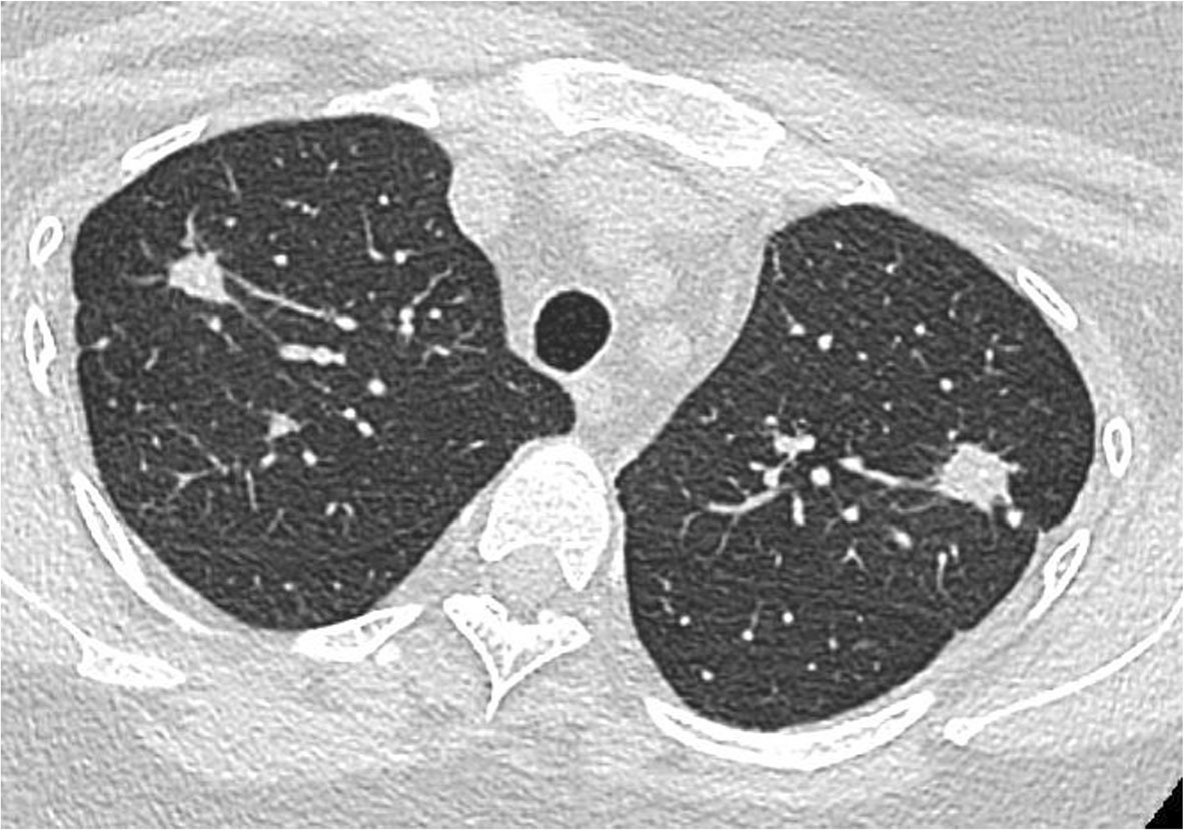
CT Chest of patient showing multiple granulomas
